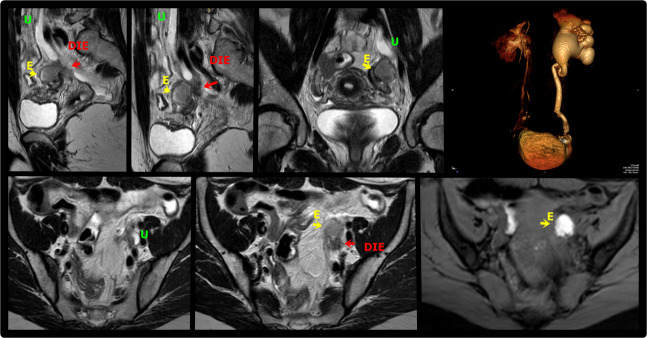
Sagittal (A, B), coronal (C) and axial (E,F) T2W and axial T1W with fat saturation (G) MR images demonstrate deep endometriosis thickening of the left paracervical area(arrow) involving the parametrium and extending to the ovarian fossa and adjacent ureter, resulting in upstream hydroureter and hydronephrosis. There is also an endometrioma in the left ovary (E). MR urogram volumetric reconstruction shows asymmetry of the ureters with accentuated hydronephrosis to the left (D). DIE: deep endometriosis, U: Ureter, E: Endometrioma.
